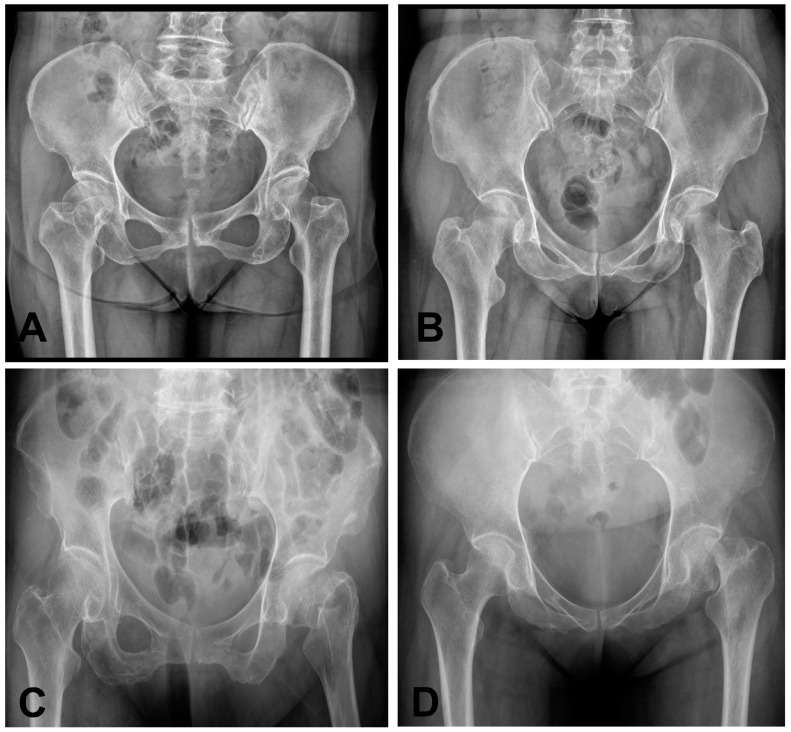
Representative radiographs of different femoral neck fracture cases. (A,B) Shows radiographs of nondisplaced femoral neck fractures (Garden I/II), while (C,D) are displaced femoral neck fractures (Garden III/IV).
